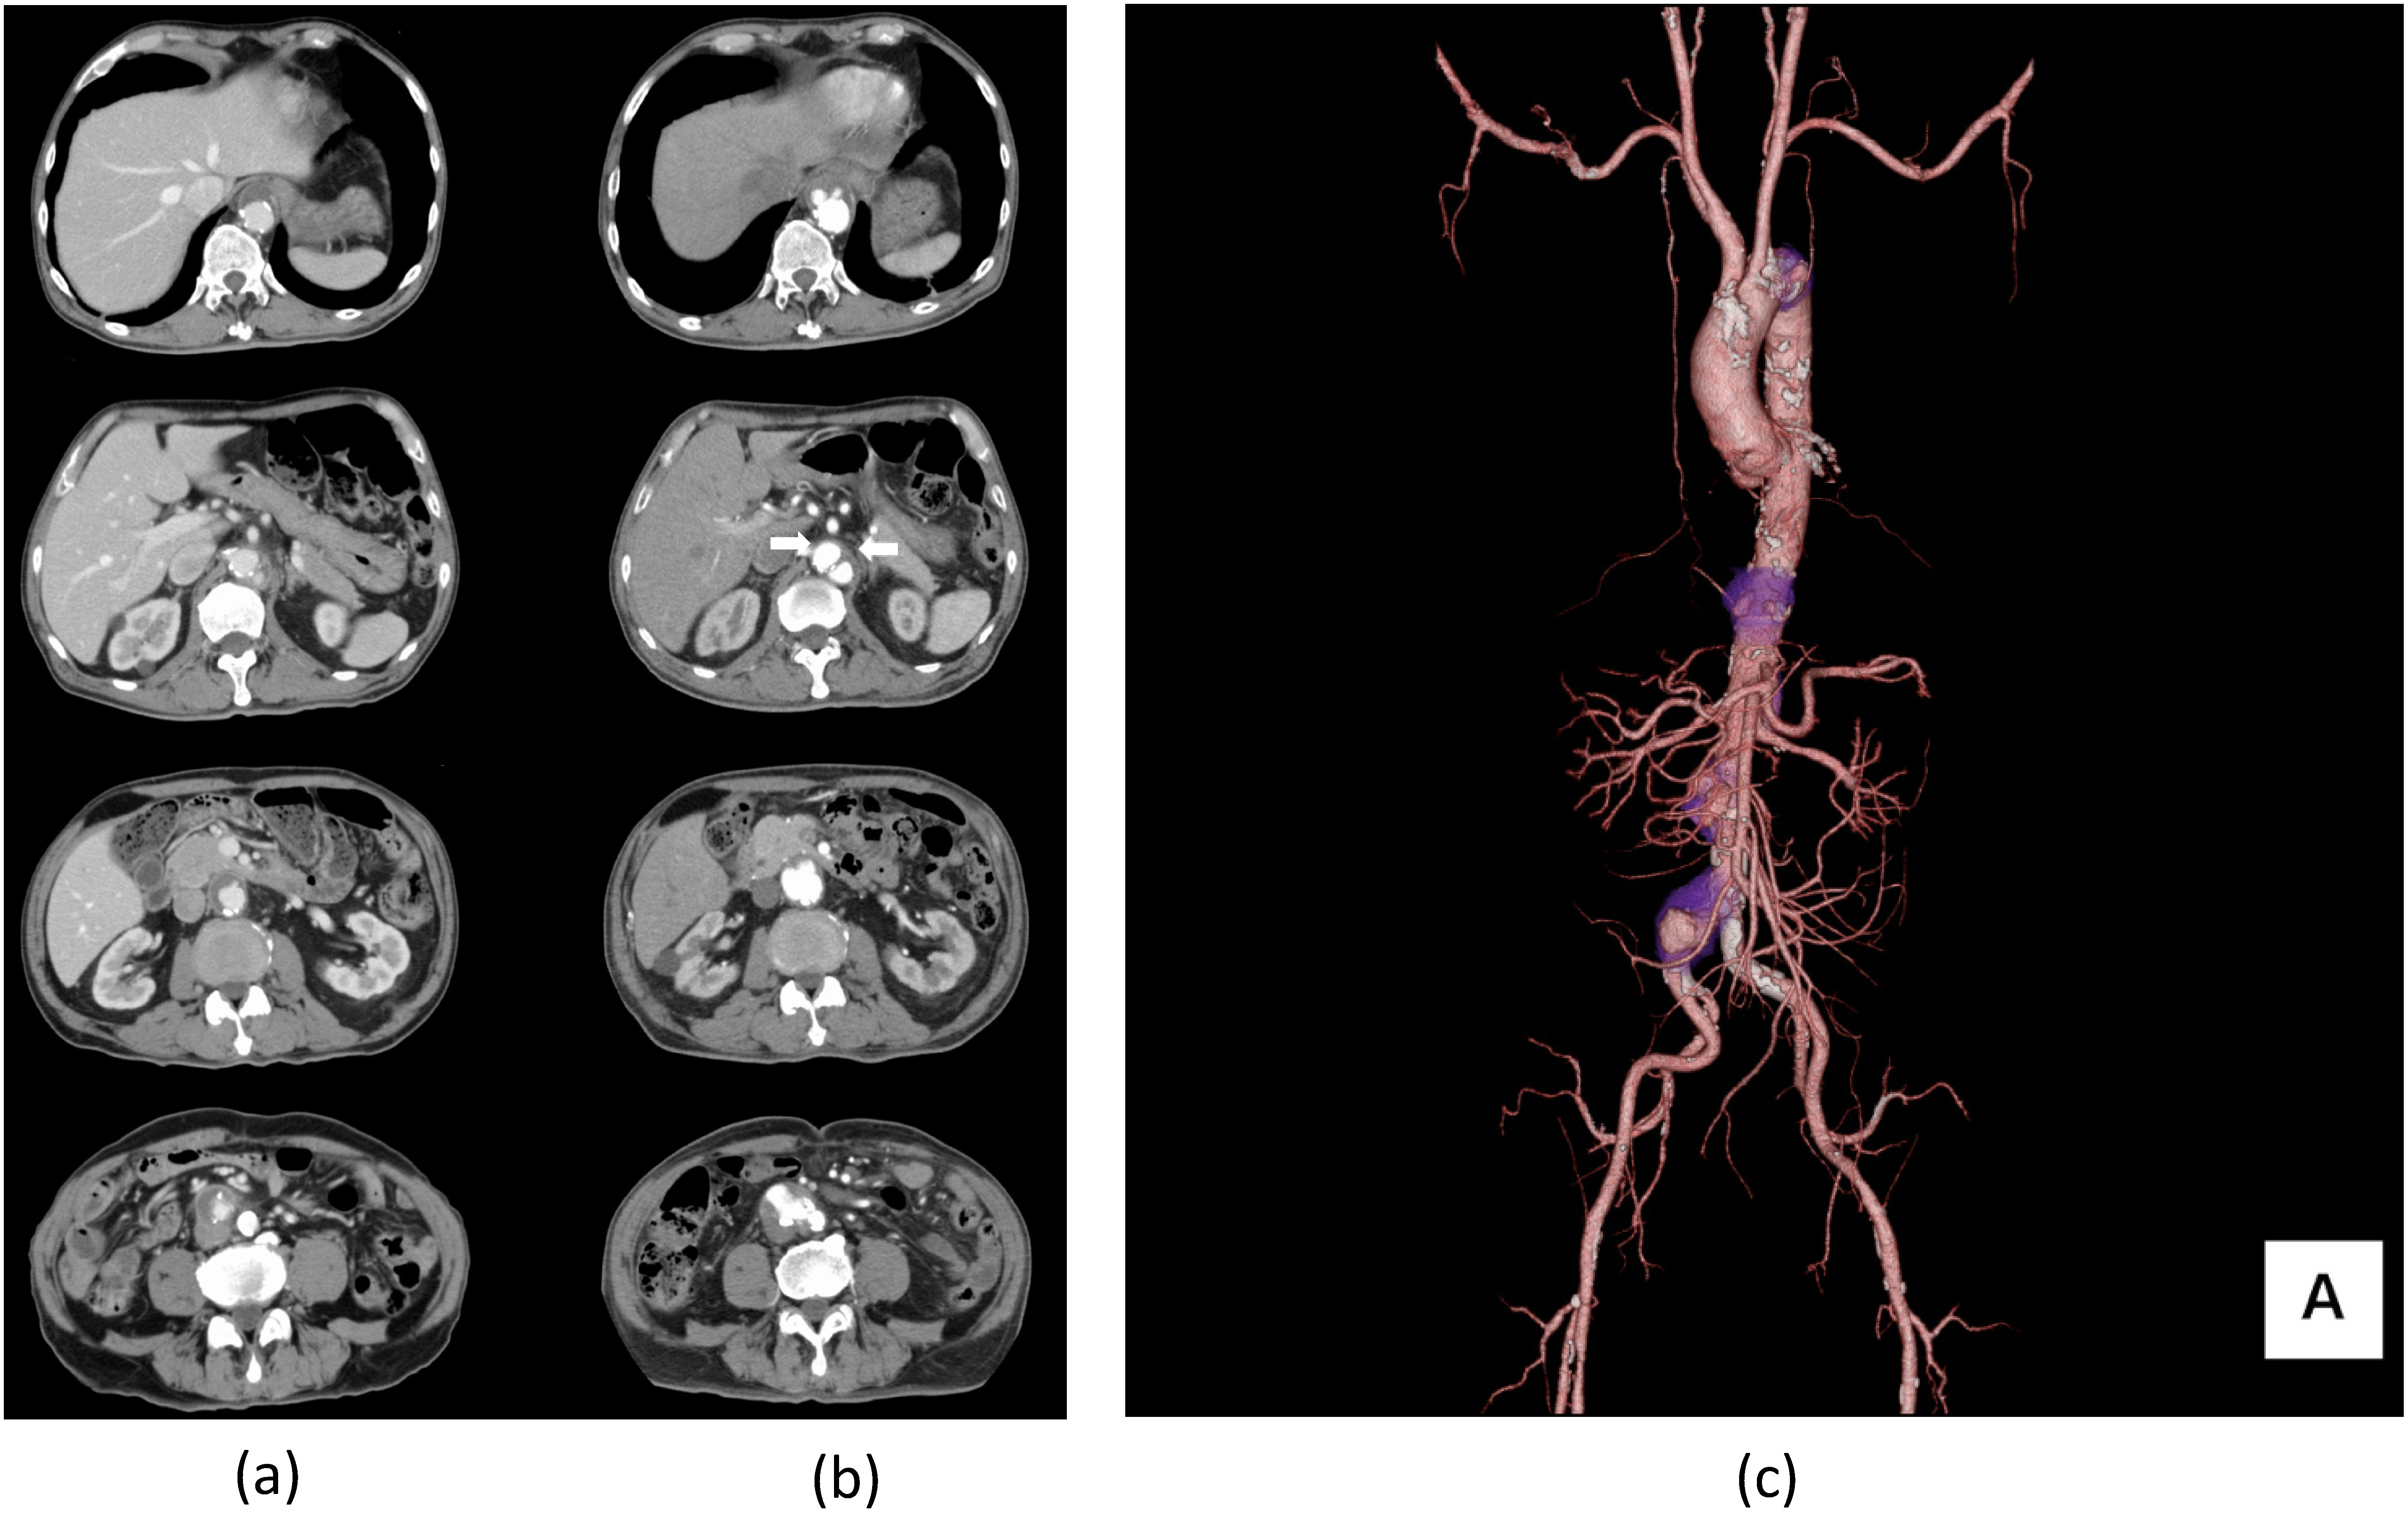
Fig. 1 Contrast-enhanced computed tomography (CT) examination at admission (a) and one month later (b) and (c) show multiple aortic aneurysms and rapid enlargement of the aneurysm diameter. The portion corresponding to the sealing zone between the fenestration of the celiac artery and the superior mesenteric artery and the saccular aneurysm is indicated by arrows.
